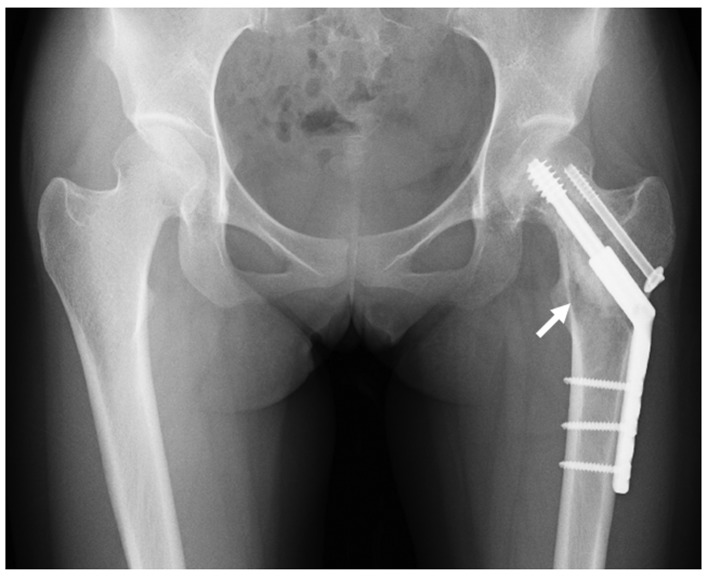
Radiographic observations following the first surgery. The tumor has been curettaged and hydroxyapatite (HA) granules have been transplanted (arrows).
